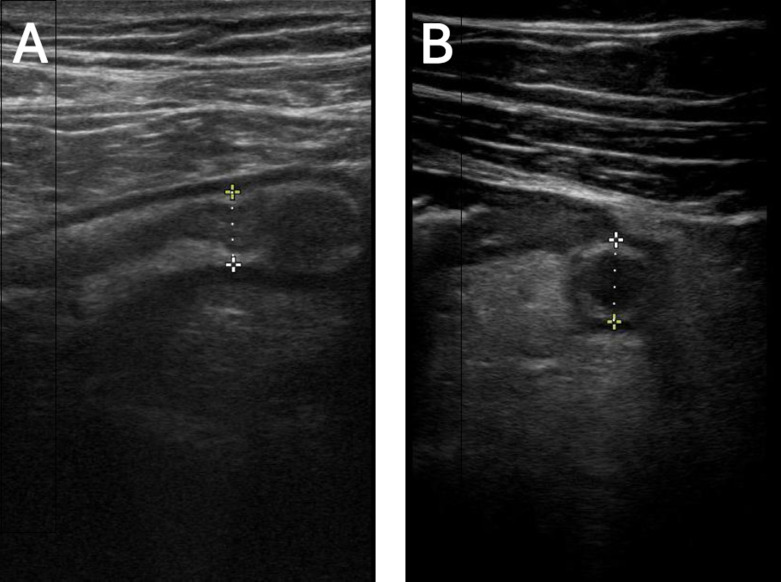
Point of care ultrasound (POCUS) findings with anterior-posterior appendiceal diameter greater than 6mm in longitudinal (A) and transverse (B) views.
